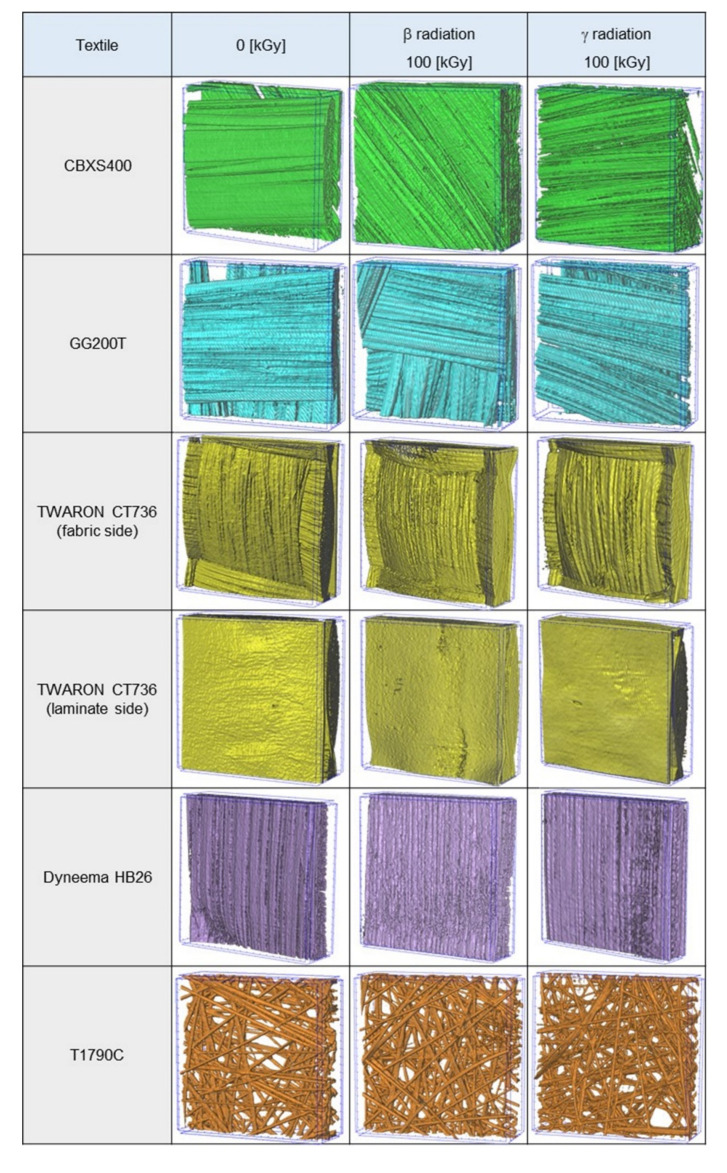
Three-dimensional micro-computed tomography reconstructions of unmodified and irradiated textiles (surface area 2 mm × 2 mm).
